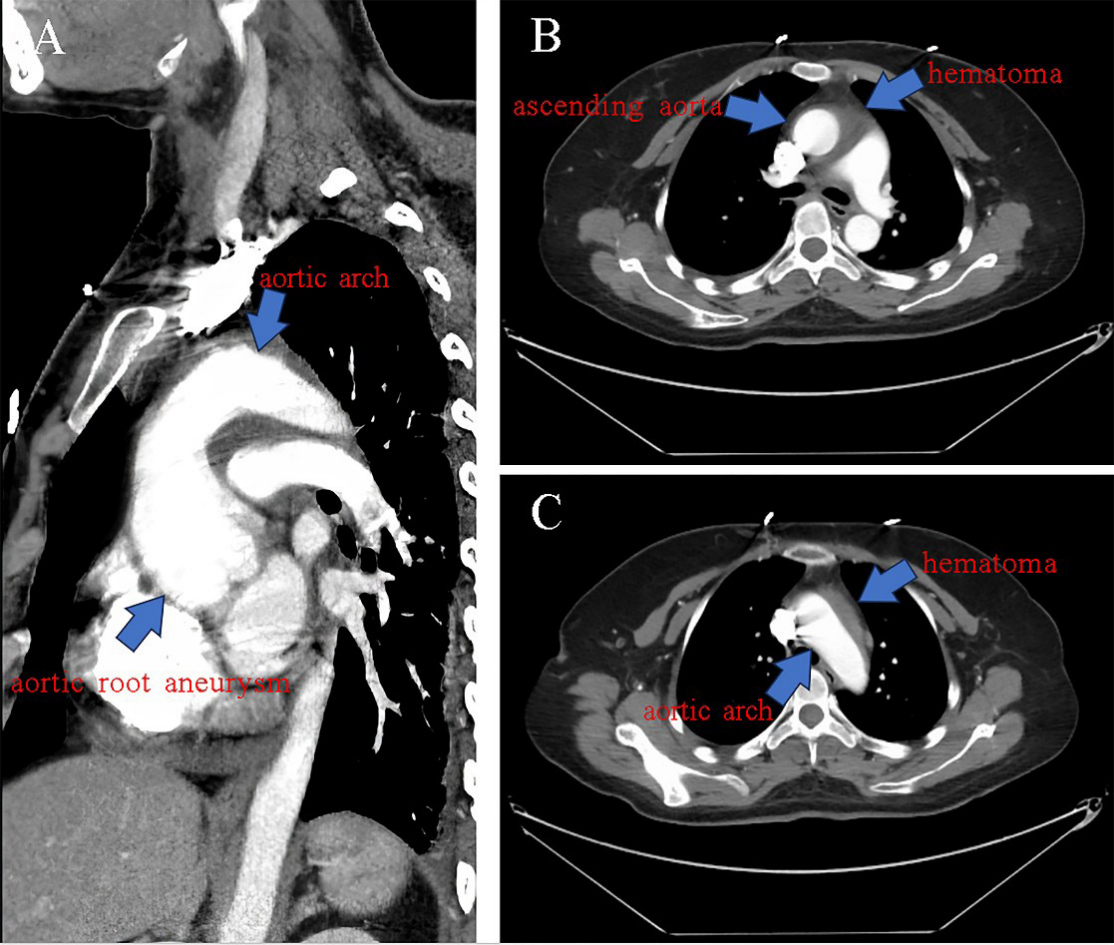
Computed tomography angiography images. A) Aortic root aneurysm (arrow).
